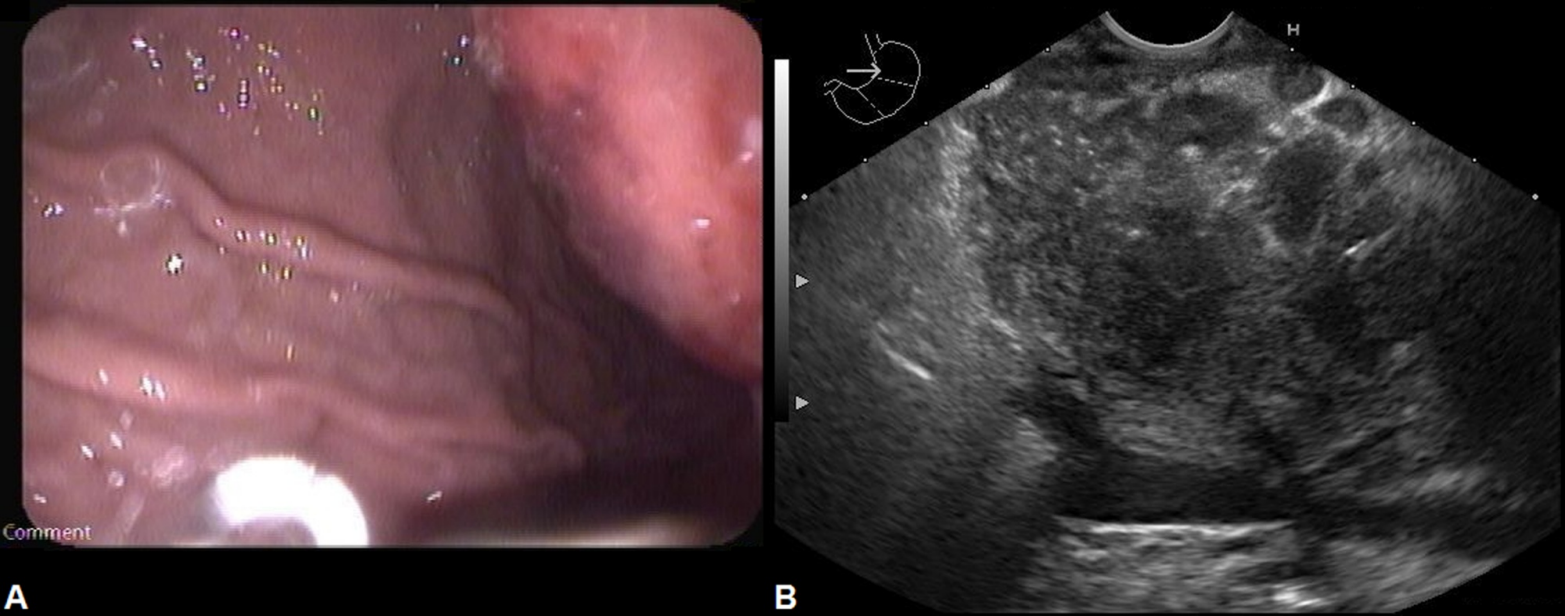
Gastroscopy with endoscopic ultrasound (A) Gastroscopy shows an extrinsic mass causing compression and visible indentation within the lumen of the stomach. (B) Image of endoscopic ultrasonography demonstrating an ill-defined necrotic mass within the pancreas.
